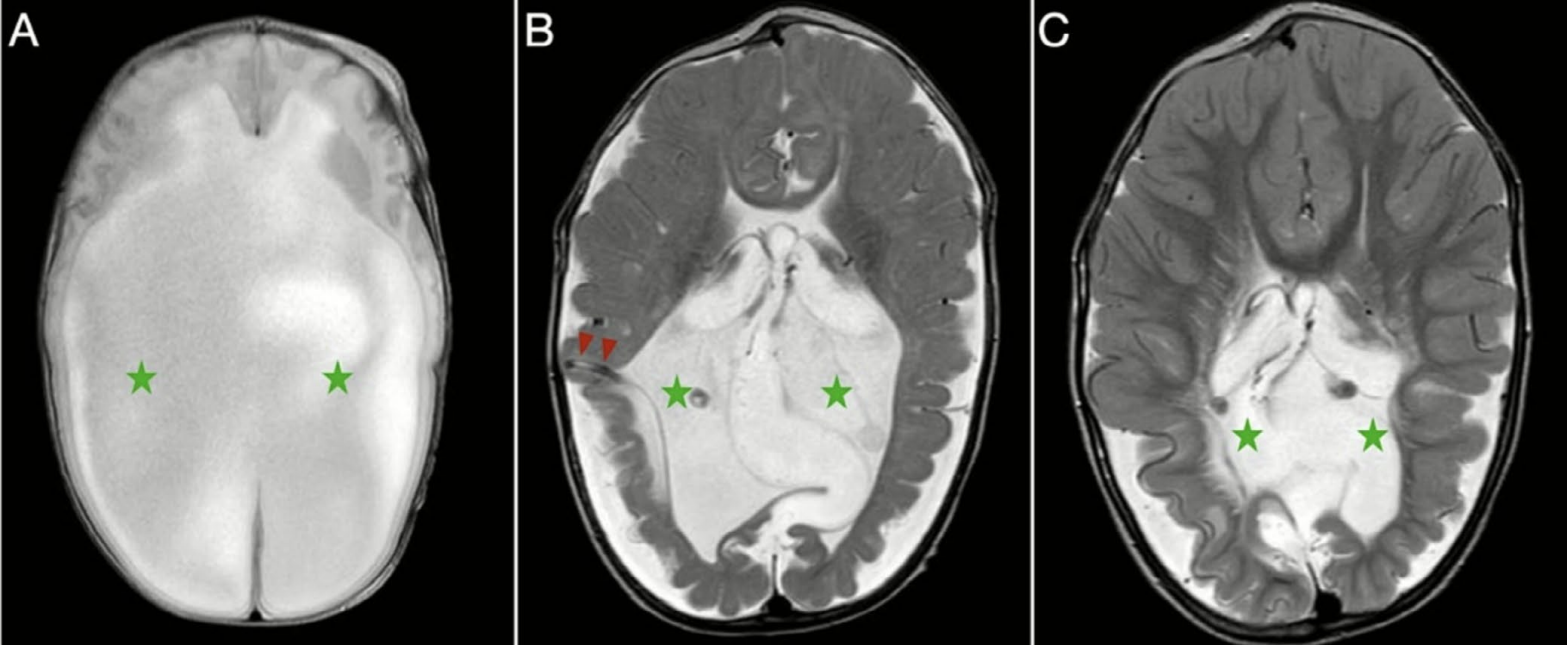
Serial axial T2‐weighted MRI images of the brain—(A) at presentation, (B) following insertion of a ventriculoperitoneal (VP) shunt (burgundy arrowheads), and (C) on two‐year follow‐up—demonstrate progressive reduction of hydrocephalus (green stars).
